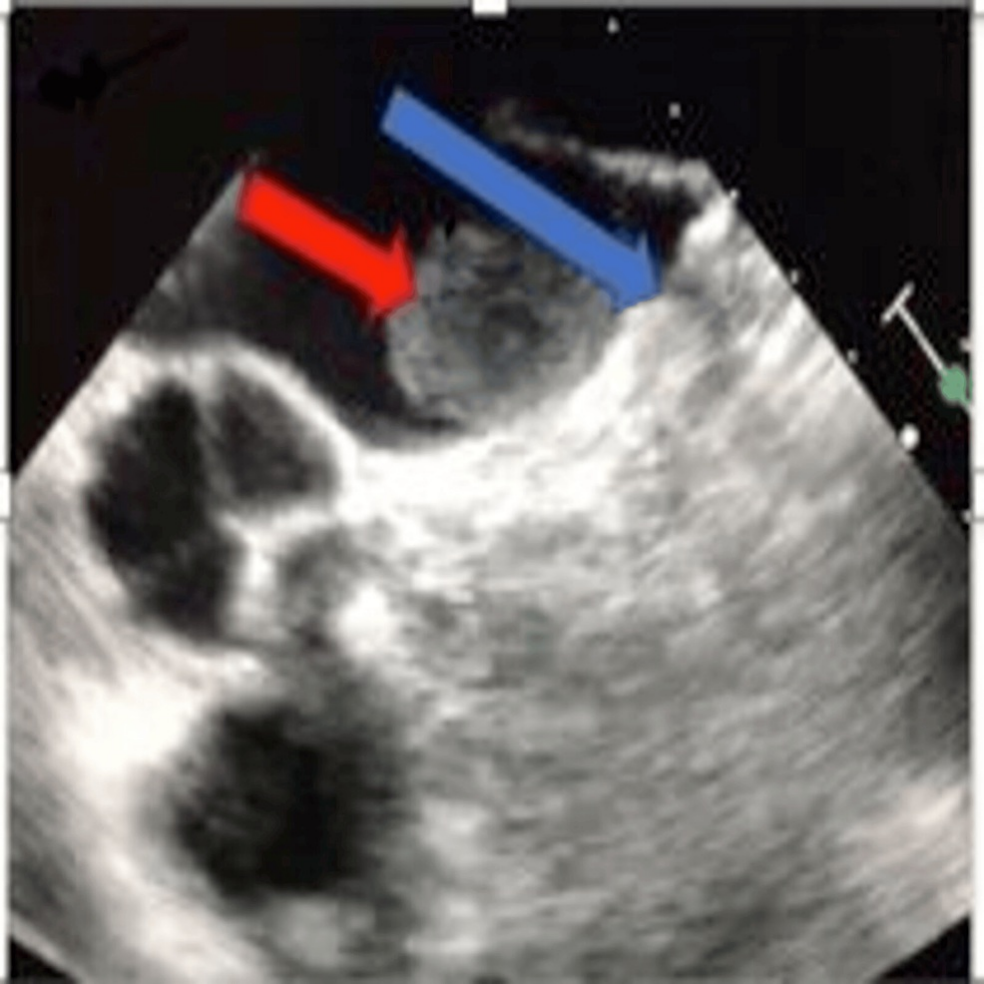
Mid-esophageal AV view of a thrombus formation as seen on TEE 49 days post-implantation of the WATCHMAN device. The red arrow indicates a thrombus, and the blue arrow points to the WATCHMAN device. AV: aortic valve; TEE: transesophageal echocardiogram
